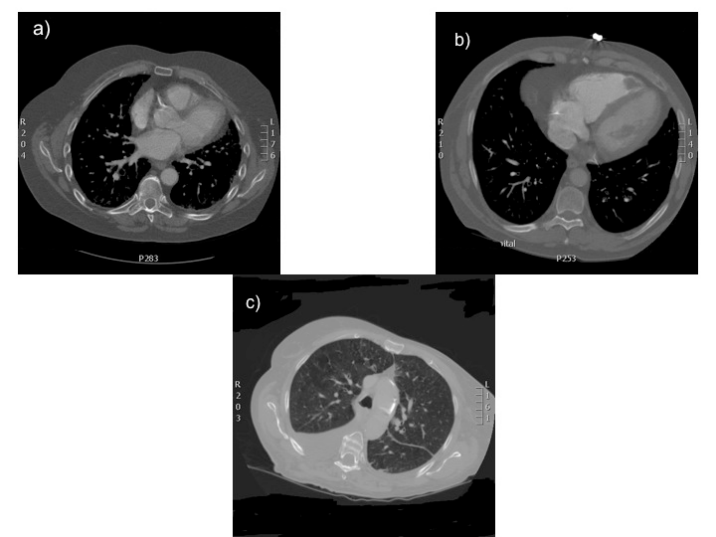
Examples of incidental findings observed on CT pulmonary angiograms. Figure 1a: coronary calcification, Figure 1b: right ventricular mural thrombus. Figure 1c: aortic wall calcification.
